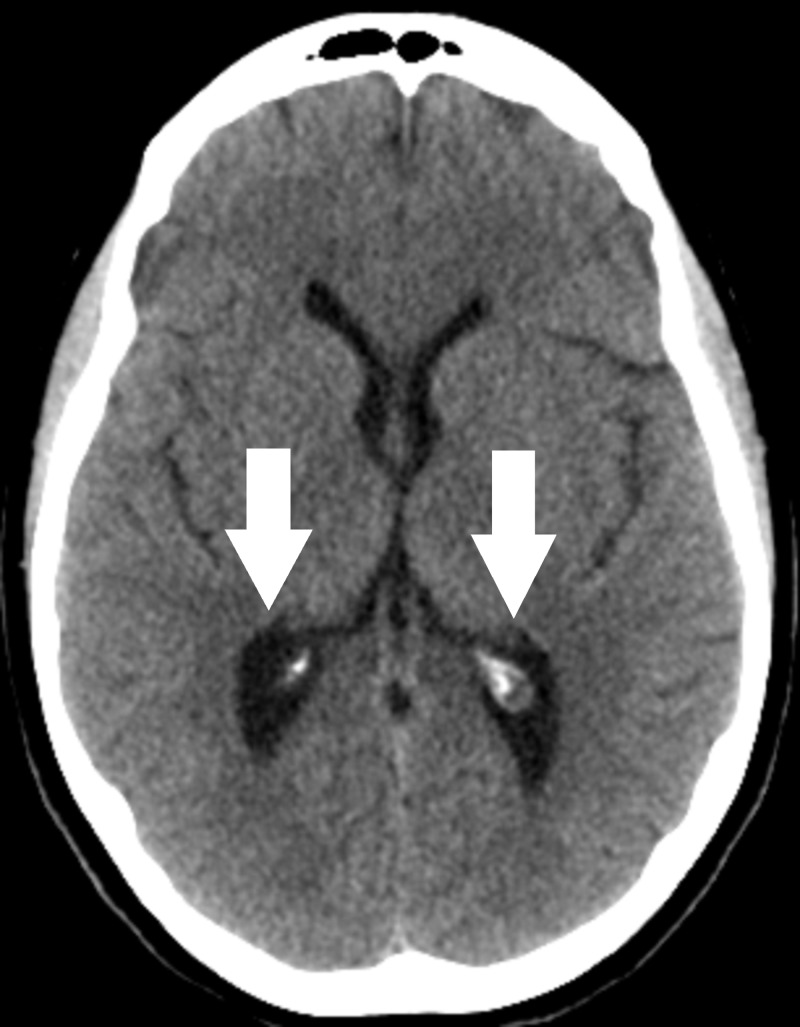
Axial computed tomography (CT) following endoscopic third ventriculostomy. Note unchanged size of lateral ventricles.
